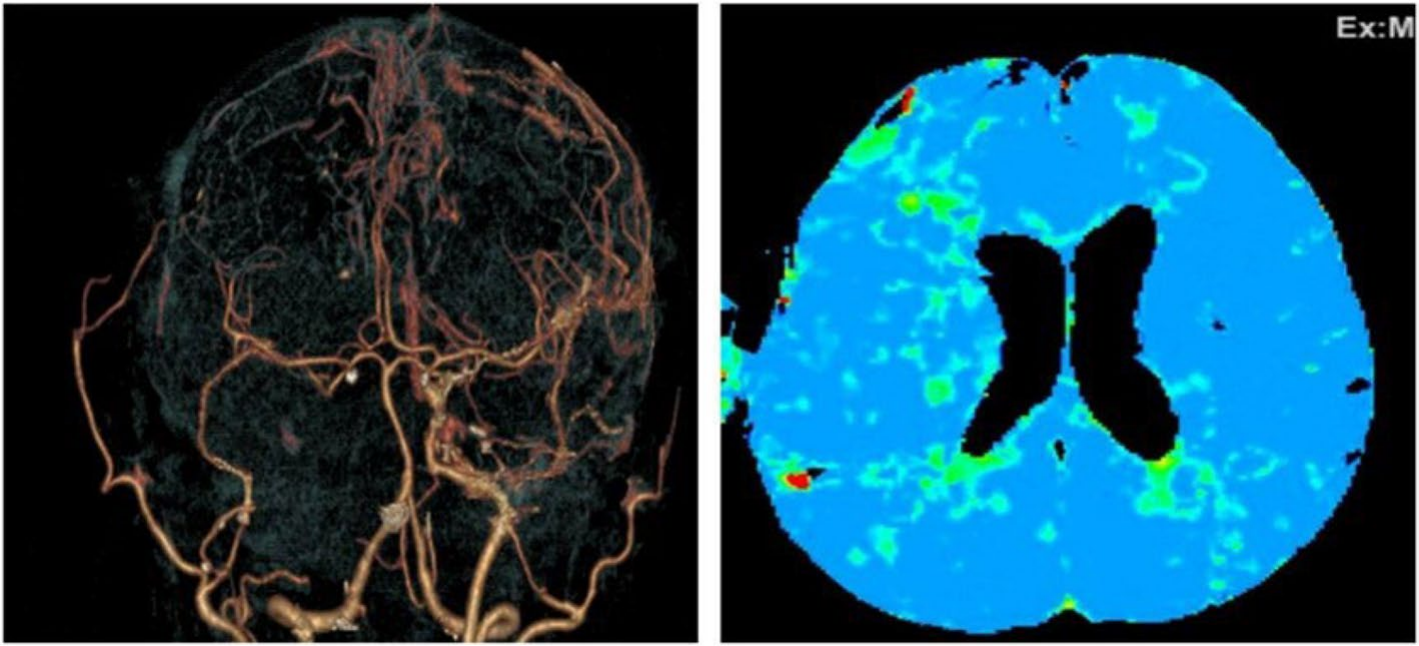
Post-operative CTA and CT perfusion
